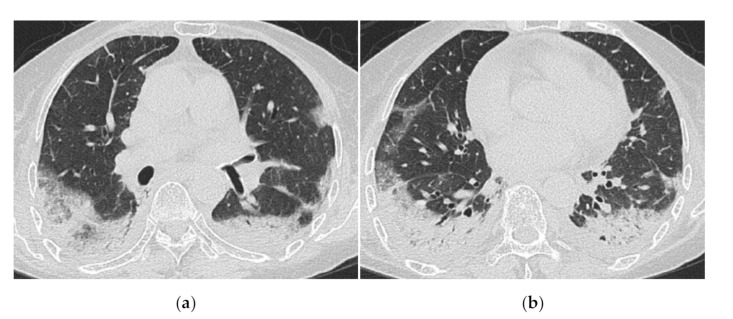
A 70-year-old woman with rapidly progressive interstitial lung disease associated with clinically amyopathic dermatomyositis (antibody unknown). HRCT images (a,b) show diffuse ground-glass opacification and consolidation just below the pleura. Crazy-paving appearance, interlobular septal thickening and bilateral pleural effusions are also seen. The patient died 2 weeks after admission.
